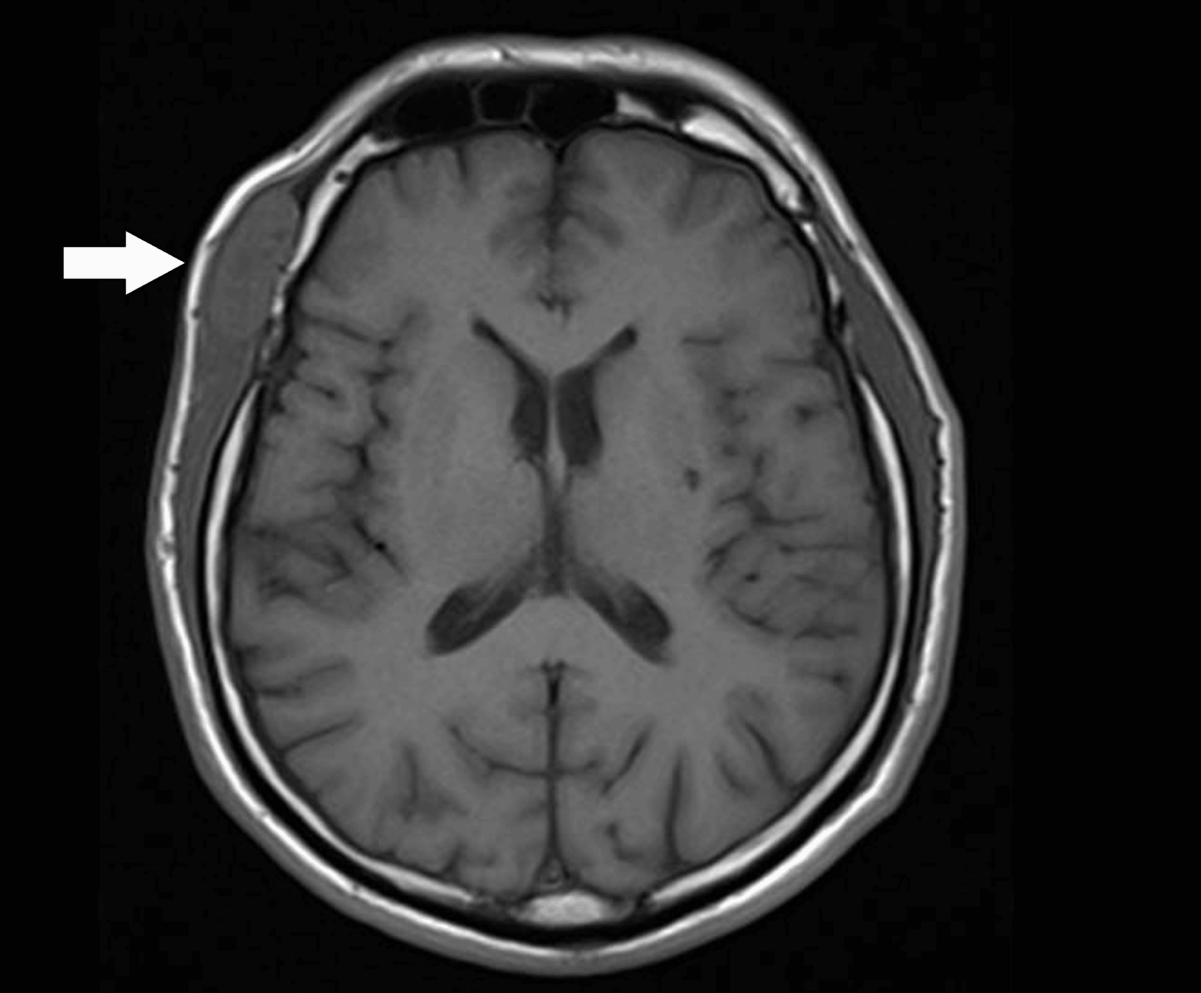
Axial-view T1-weighted image showing a right temporalis muscle lesion
